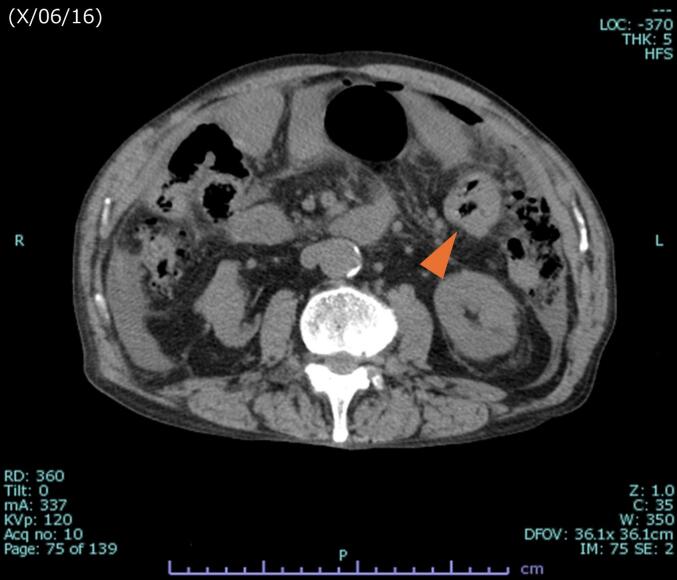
CT imaging at admission. Contrast-enhanced CT revealed thickening of the sigmoid colon wall, increased density of the surrounding fatty tissue, and the presence of free intraperitoneal air.
